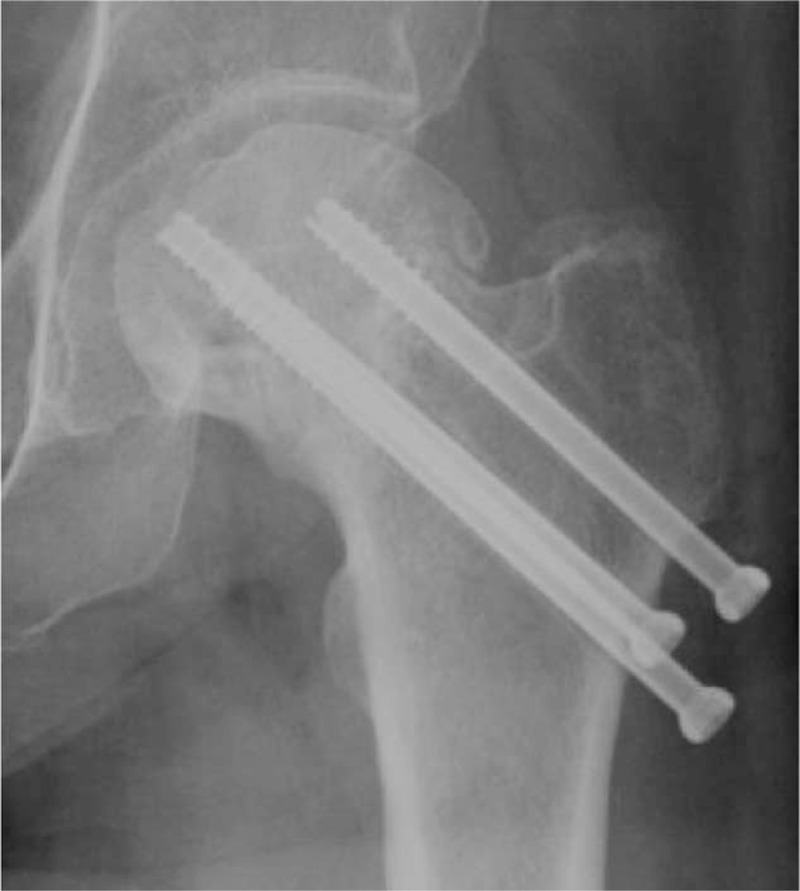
Antero-posterior radiograph at 3 years postoperatively showing the ONFH.ONFH = osteonecrosis of femoral head.
